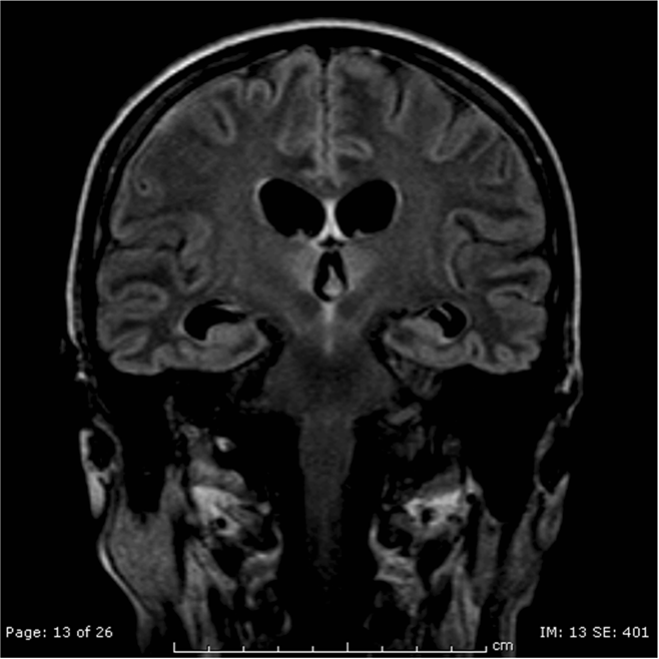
FLAIR coronal shows hyperintensity of the hypothalamic region and medial thalamus.
